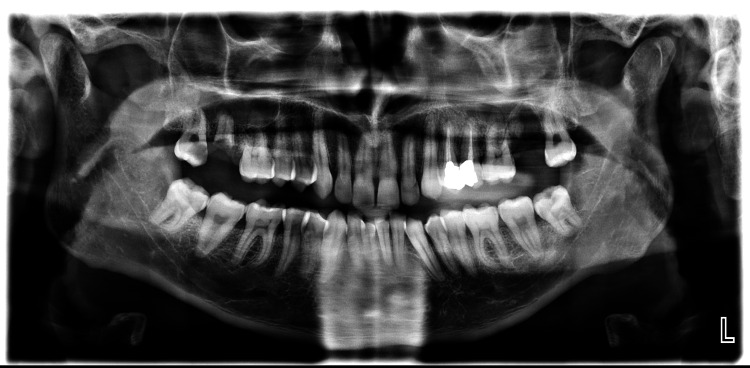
Preoperative panoramic radiograph (Case 2)
